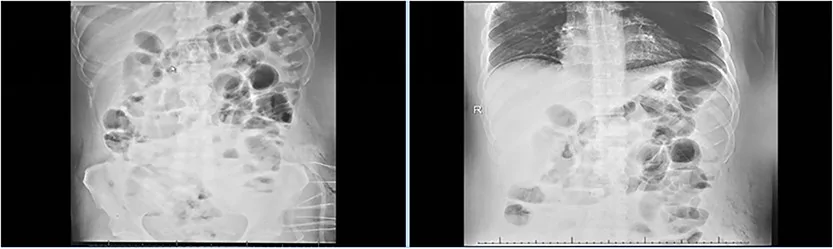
Post-operative abdominal X-ray showed improvement in the dilation.
